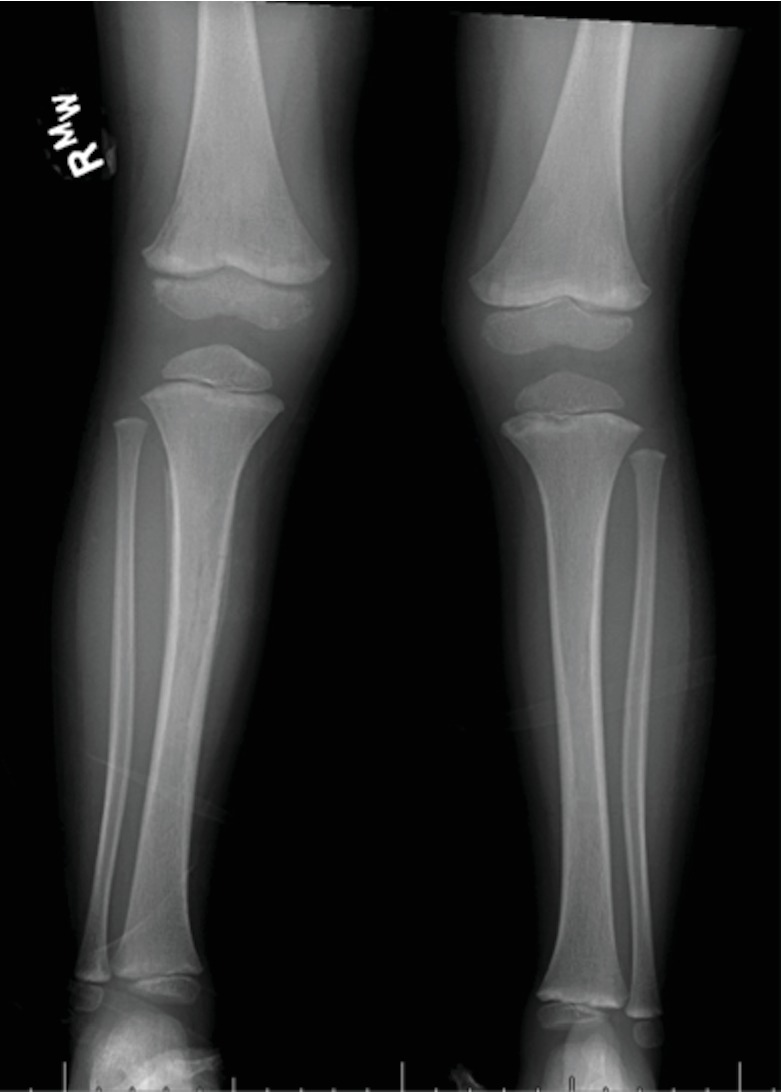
Bilateral anteroposterior plain radiograph of the lower extremities one week after initial discharge demonstrating periosteal reaction of the tibia and striated lucencies of the distal femur.
